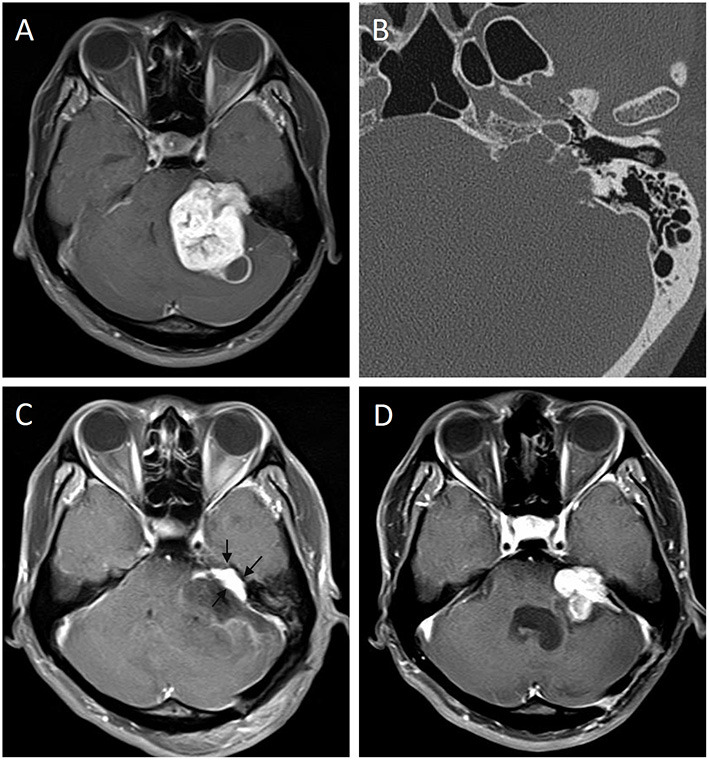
Progression of a remnant vestibular schwannoma with irregular IAC. Preoperative enhanced MRI (A) of a 29-year-old man showed a giant VS of left side. Thin-slice CT bone window image of temporal bone (B) showed irregular IAC type. Three-month postoperative enhanced MRI (C) showed remnant tumor located in the IAC (arrows). The tumor had progression 3 months later (D).
